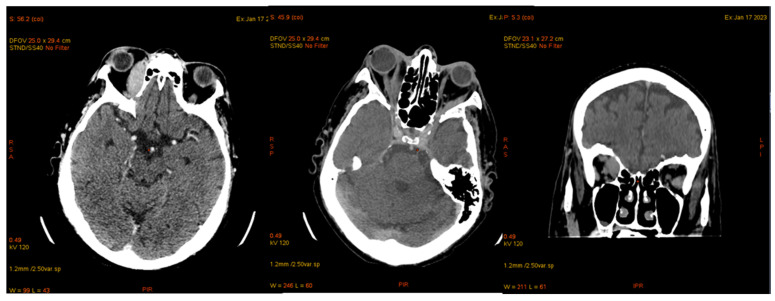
Cerebral CT—tumoral mass right and left eye.
